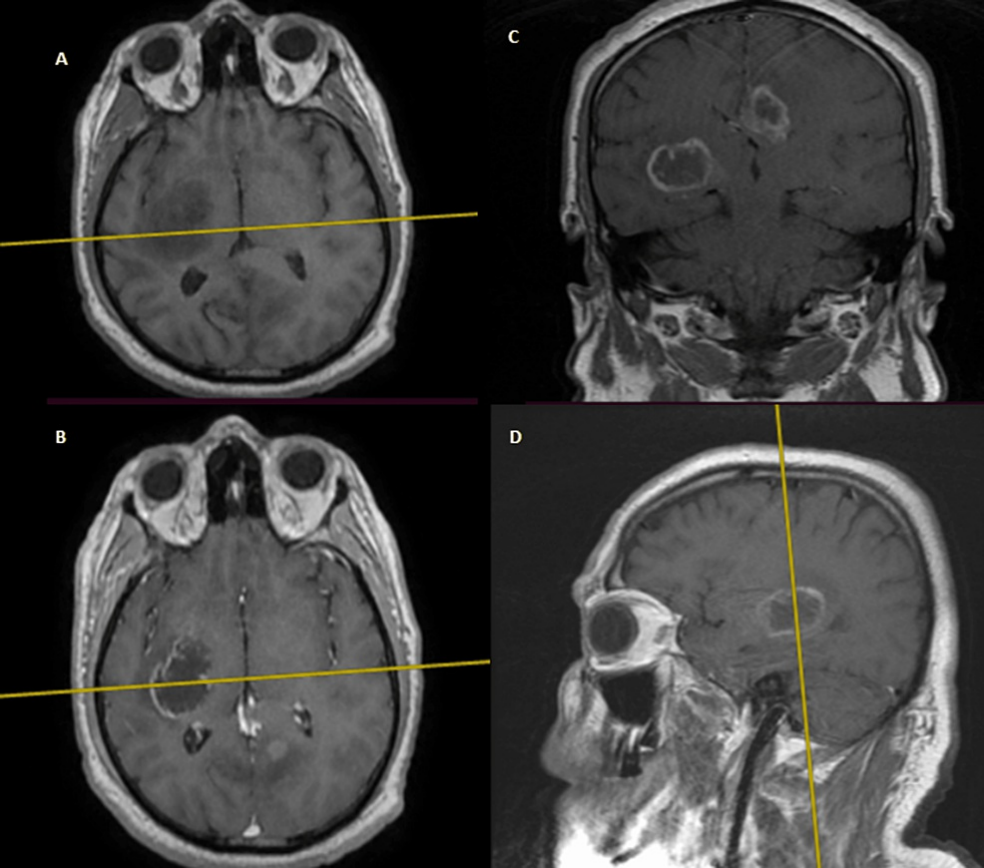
Cranial MRI showing the first lesion (insular right-sided, all T1 sequences) (A) Axial cut without contrast; (B) axial cut with contrast; (C) coronal cut with contrast; (D) sagittal cut with contrast Image courtesy: Al Zahraa Hospital University Medical Center (ZHUMC) Radiology Department
